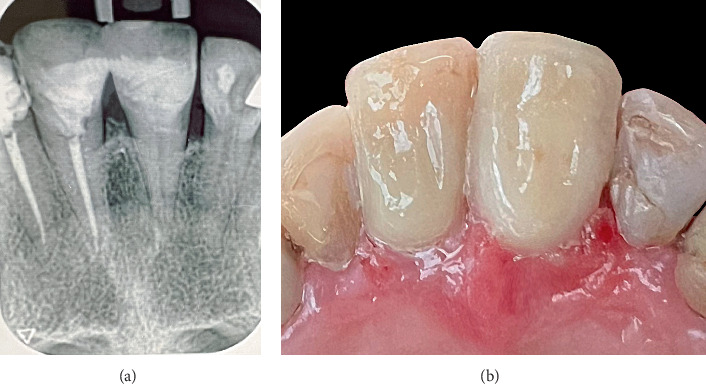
(a) Periapical radiograph of the final restoration. (b) Clinical view of the completed restoration.
